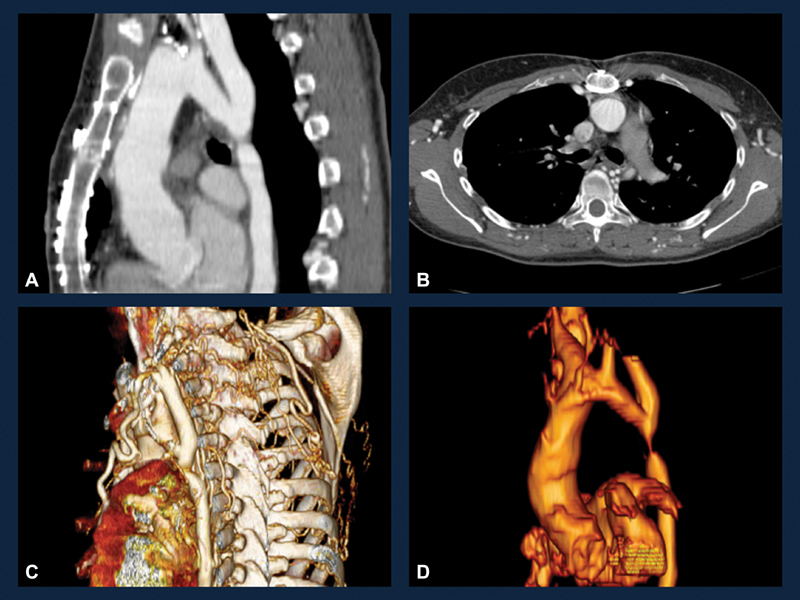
Computed tomography angiography of the chest demonstrating interrupted aortic arch. (
